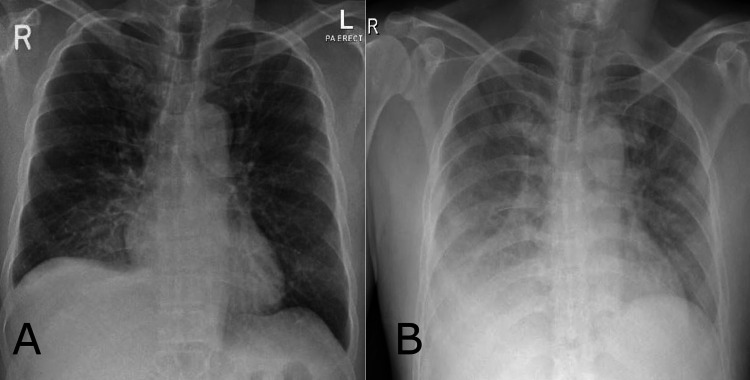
Chest X-ray: (A) prominent bronchovascular markings with ground glass opacities in the lower zone of both lungs; (B) repeated chest X-ray showing worsening reticular alveolar infiltrates involving both lungs, predominately at bases and middle quadrants.
